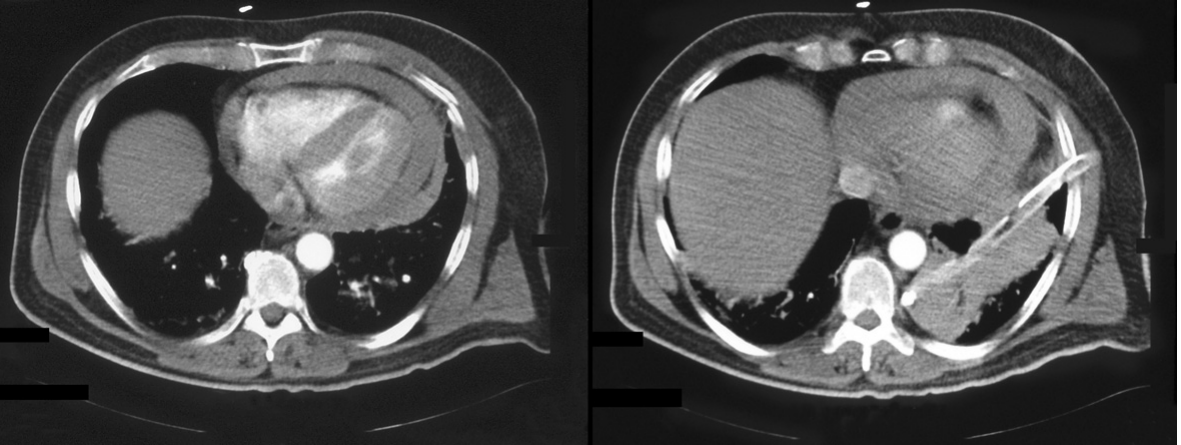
CT Scan of chest showing a) hemopericardium b) hemopericardium and residual left pleural clot and blood despite pleural drainage.
